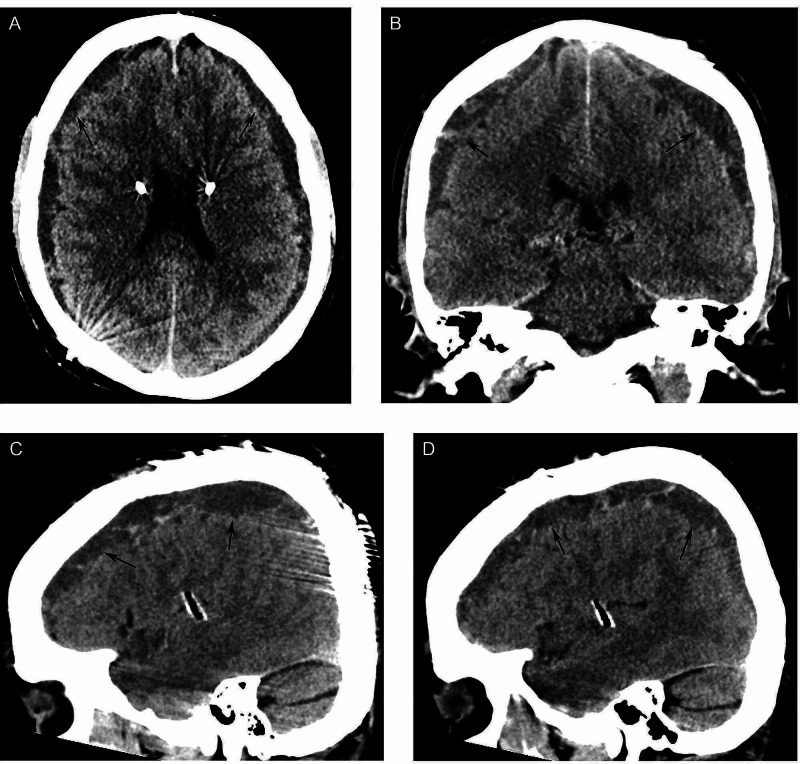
Postoperative head CT. (A) Axial, (B) coronal, (C) right para-sagittal, and (D) left para-sagittal non-contrast head CT from postoperative day 15, showing bilateral hygromas with crescentic area of hyperattenuation in the left parieto-occipital region concerning for acute to subacute subdural hematoma superimposed on layering blood products.
